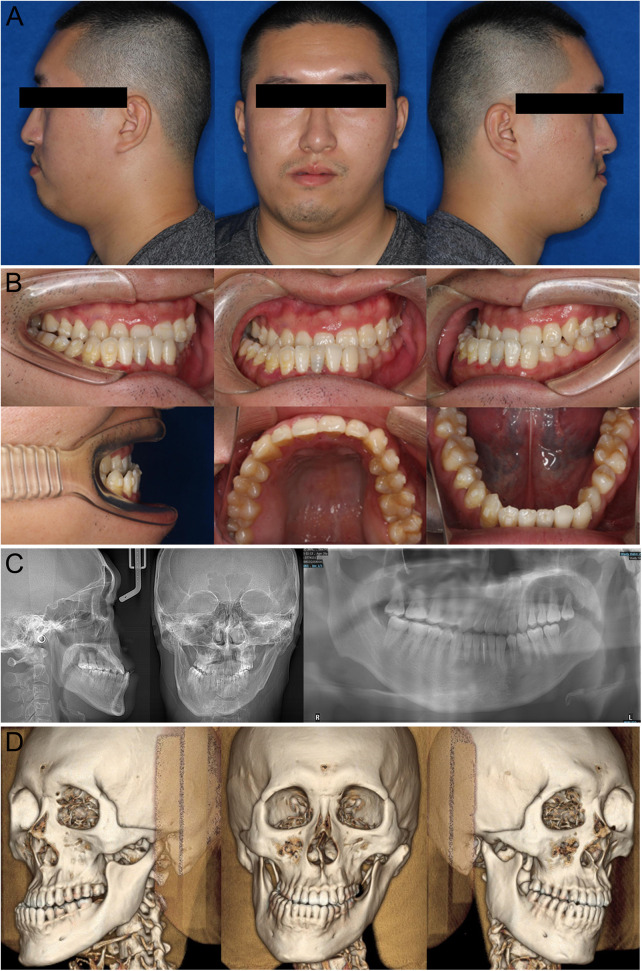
Representative patient with a chief complaint of facial asymmetry. (A) Pretreatment facial photographs show severe hyperplasia of the left mandibular body and chin deviation 15 mm to the right; (B) intraoral photos show labially inclined upper incisors, deviated lower dental midline to the right and right posterior teeth buccally crossbite; (C) pretreatment lateral and posteroanterior cephalograms as well as panoramic radiographs; (D) pretreatment CT scan shows right deviation of the chin and left mandible body significantly lengthened horizontally.
